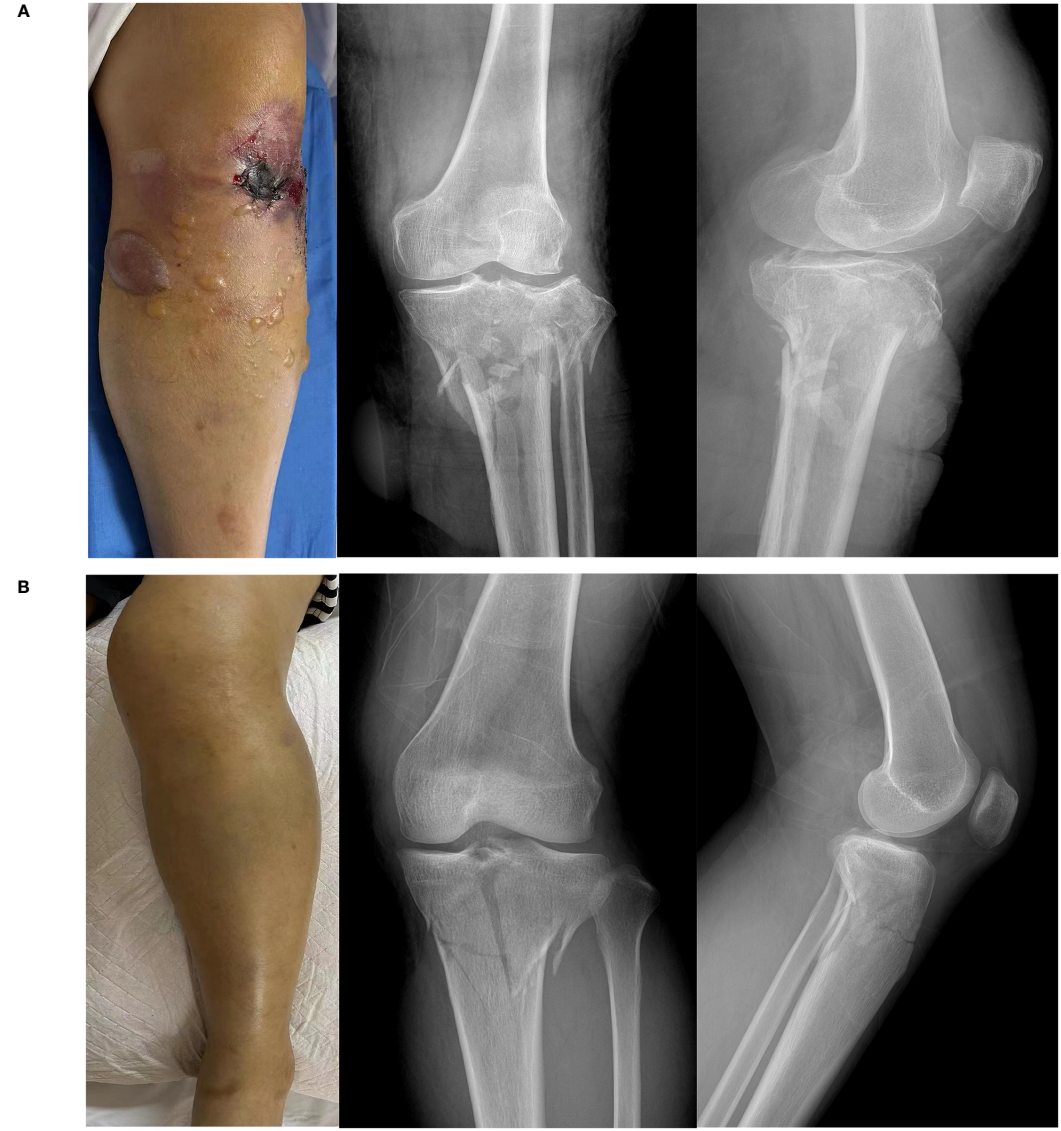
Images and X-ray of a tibial fracture. (A) Tibial fracture patient with acute compartment syndrome. (B) Tibial fracture patient without acute compartment syndrome.
